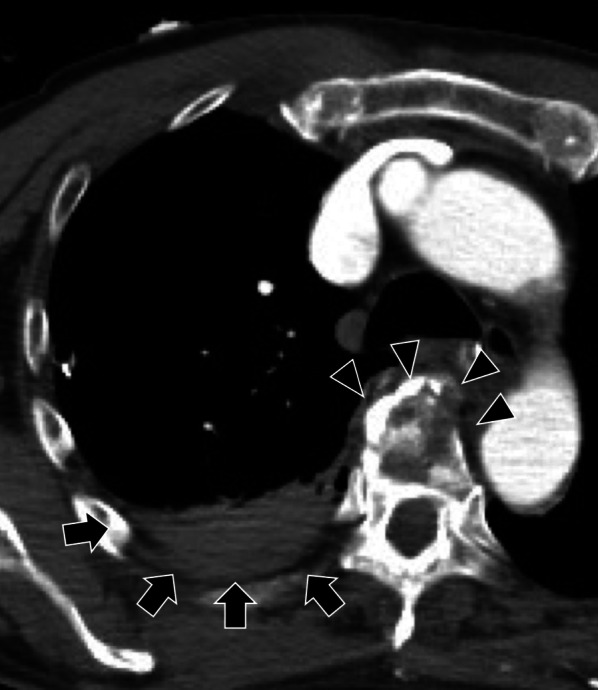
Contrast CT images showing the injuries sustained by the patient. Axial view of the chest, highlighting the fluid collection on the dorsal side of the chest cavity (*arrows*) and demonstrating the spinal body fracture of the fifth thoracic vertebra (*arrowheads*). No obvious extravasation is seen
